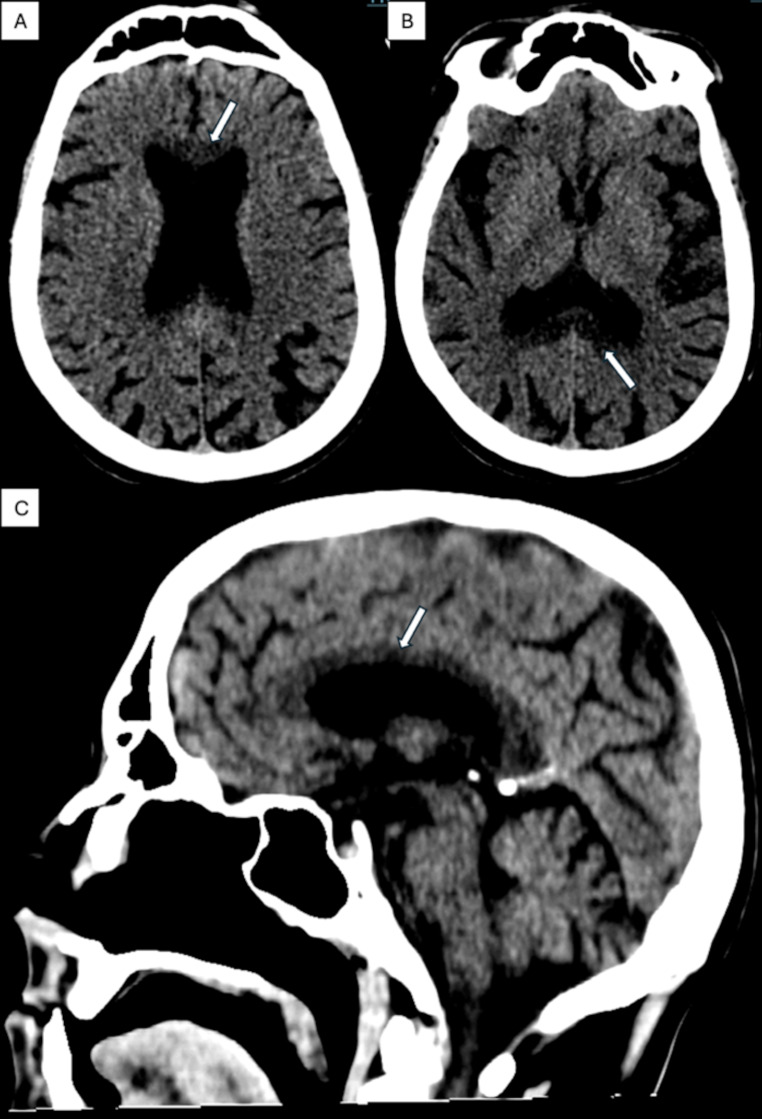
Native head CT images. Panel A: Axial CR scan, showing conspicuous hypodensity (arrow) of the genu of the corpus callosum in the area between the frontal horns of the lateral ventricles. Panel B: Axial CT scan showing the hypodensity (arrow) of the splenium of the corpus callosum in the area between the occipital horns of the lateral ventricles. The symmetrical narrowing of the gyri and widening of the sulci along the convexity of the hemispheres are in keeping with the diagnosis of generalized brain atrophy. Panel C: Sagittal CT scan showing the marked hypodensity (arrow) and thinning of the corpus callosum at the top of the lateral ventricle
